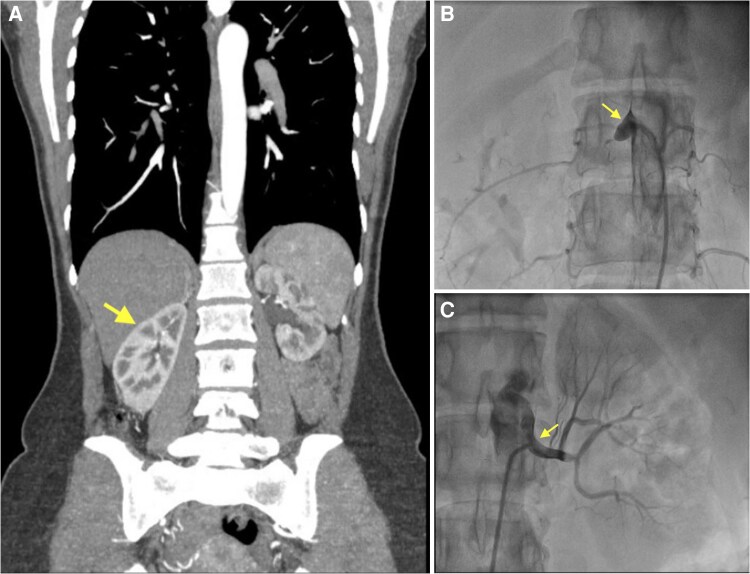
(A)* Contrast-enhanced computed tomography demonstrated patency of the left renal artery and total occlusion of the right renal artery. Additionally, computed tomography findings confirmed preserved renal size, with no radiological evidence of renal atrophy. *(B)* Conventional angiography demonstrated total occlusion of the right renal artery beginning at its proximal segment. *(C)* Conventional angiography demonstrated patency of the left renal artery.
